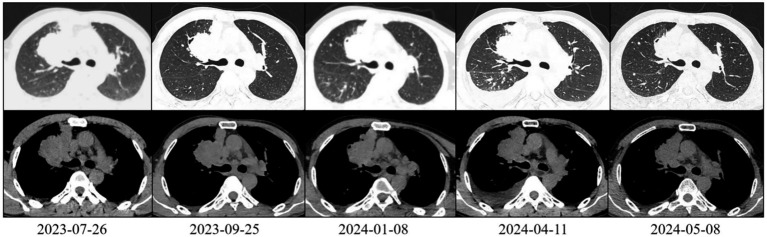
Chest CT scan of the patient (lung and mediastinal windows).
